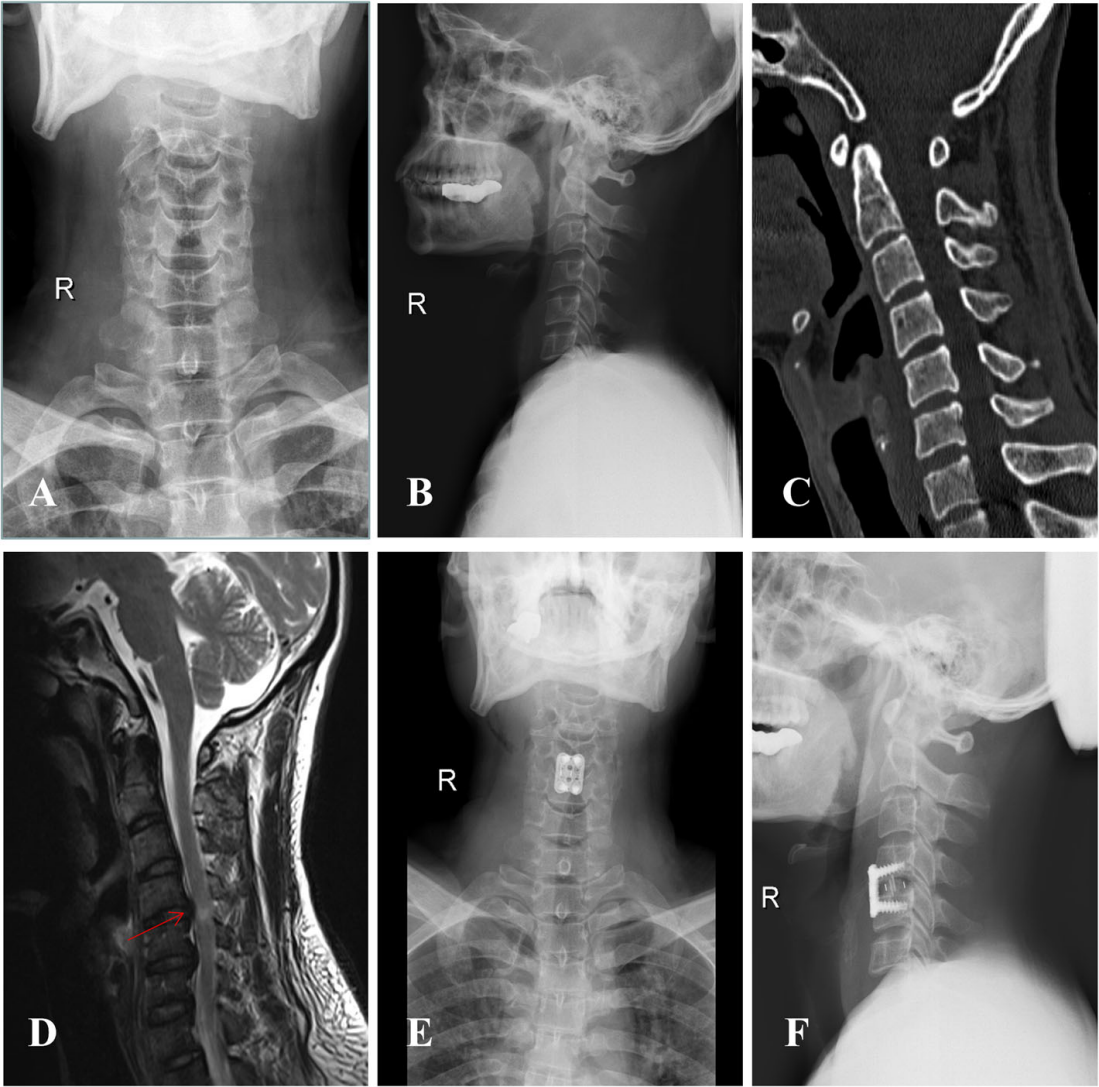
Imaging findings and operative radiographs for patient with cervical spinal cord injury without fracture and dislocation. a and b Preoperative cervical spine X-ray images with no obvious abnormal findings; c preoperative cervical computed tomography indicating no fracture or dislocation at the C4/5 segment; d preoperative cervical MRI, with the red mark showing cervical disc herniation, epidural spinal cord compression, and an abnormal spinal cord signal at the C4/5 segment; e and f postoperative cervical spine X-ray images showing cage and fixation devices in good positions after surgery
